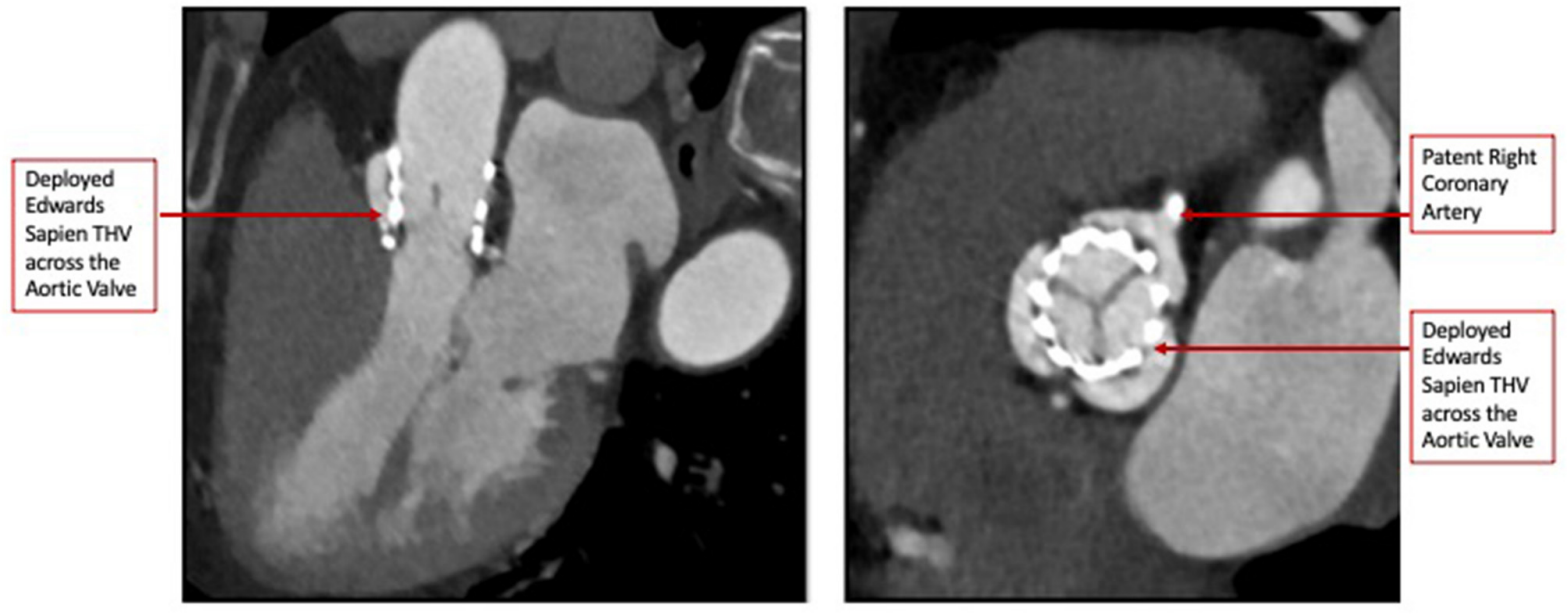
Post-TAVR Cardiac Computed Tomography images (Short and Long-axis) demonstrating thin mobile leaflets with no hypoattenuation.
